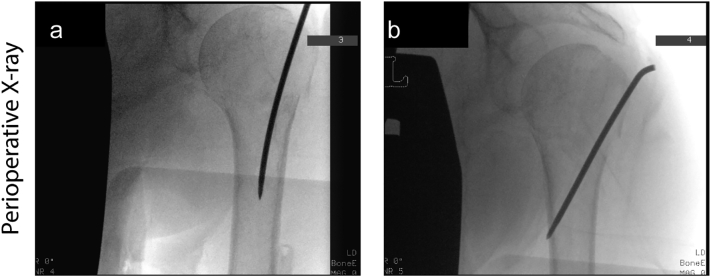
Intraoperative X-rays after closed reduction and percutaneous fixation with a single threaded K-wire.
